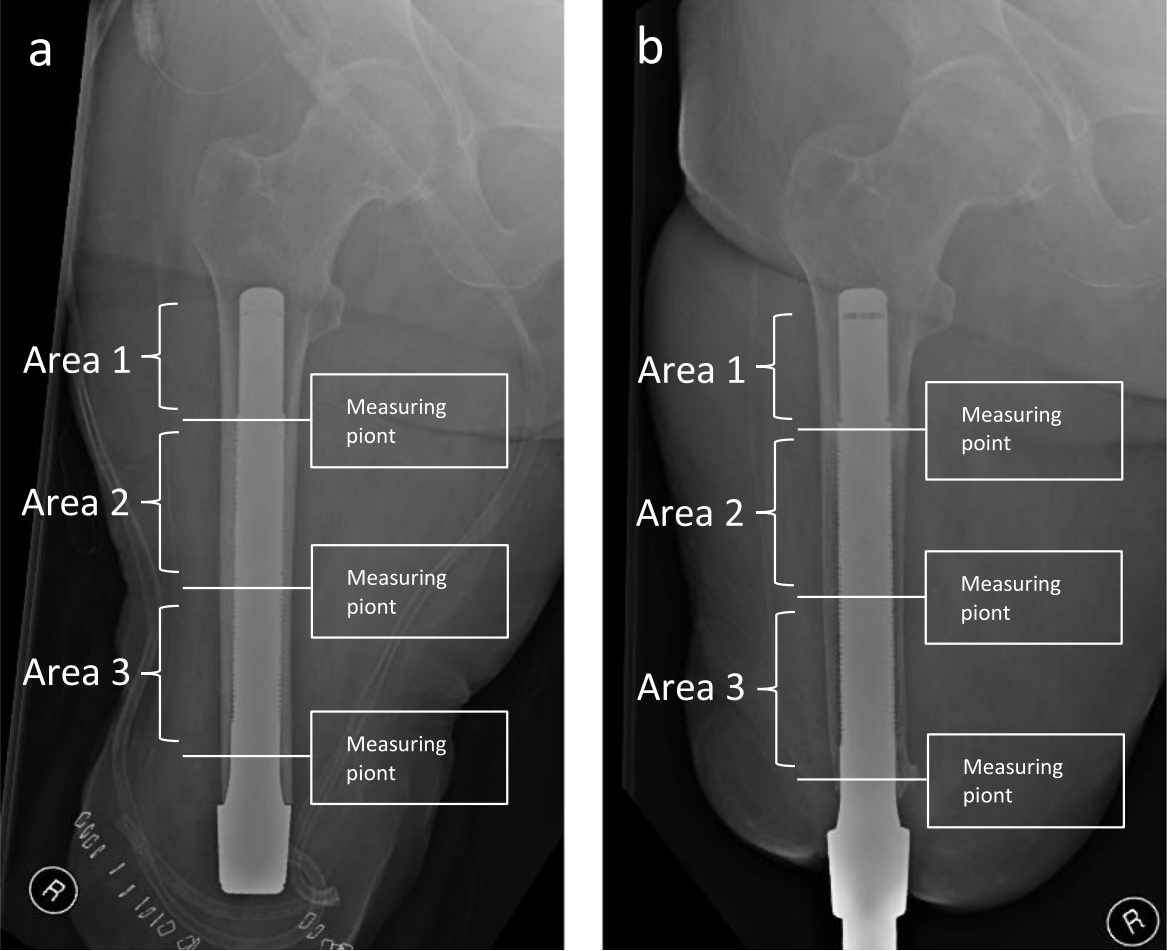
a, b Postoperative x-ray after implantation of an Endo-Fix-Stem, right thigh, and residual femur; 3-year follow-up after stem implantation, with medial and lateral atrophy of the distal femur. Additionally, a and b show the division of the femur into three areas
